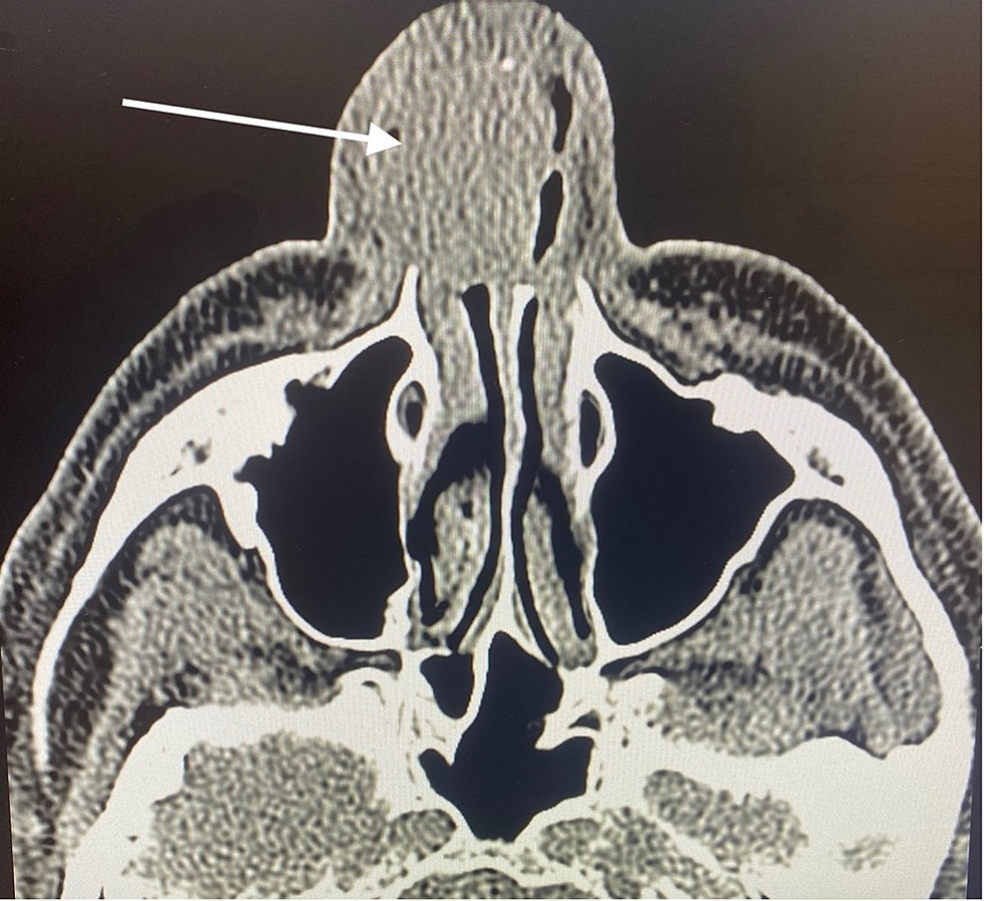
Axial CT scan without contrast at the level of the maxillary sinus in soft tissue window depicting the erosive, right sided nasal mass arising from the cartilaginous septum (arrow).
